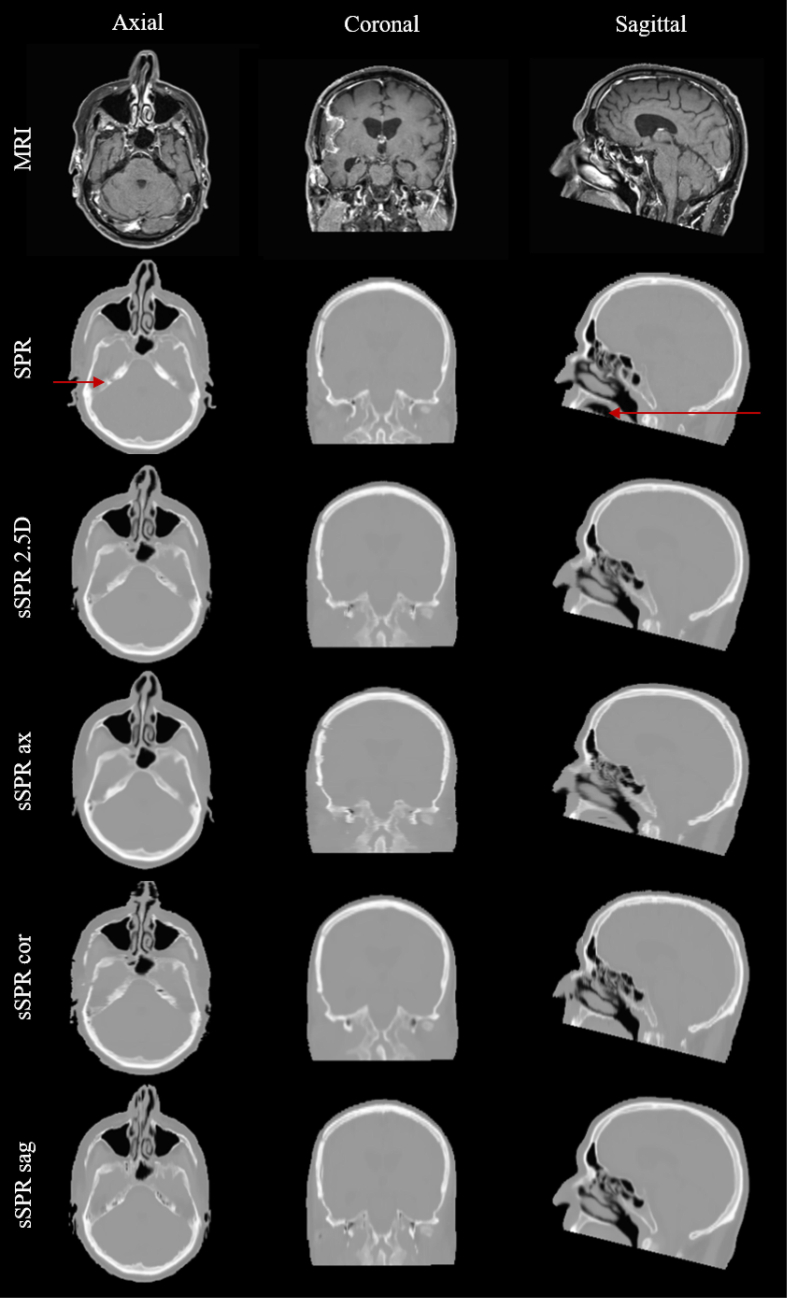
Axial, coronal, and sagittal views of patient 14. Rows show the contrast-enhanced T1-weighted magnetic resonance imaging (MRI) scan, original stopping power ratio (SPR) map, and the synthetic SPR (sSPR) maps obtained from the four approaches. The red arrows highlight regions from the original SPR that were incorrectly reconstructed in the sSPR. Window width/level: [1735 HU* / -156 HU*]. (For interpretation of the references to colour in this figure legend, the reader is referred to the web version of this article.)
